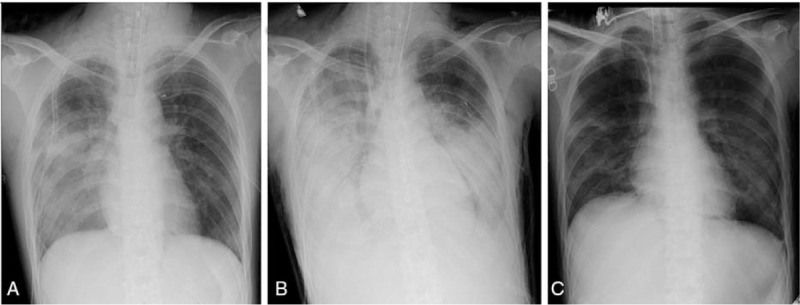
The chest radiograph on the 4th day after injury (A) showed progressive bilateral pulmonary infiltrate compared to the chest radiograph on the day of injury (B). On the 17th day after the injury (8th day after the osteosynthesis), the infiltrative shadow improved, and venovenous extracorporeal membrane oxygenation was withdrawn (C).
